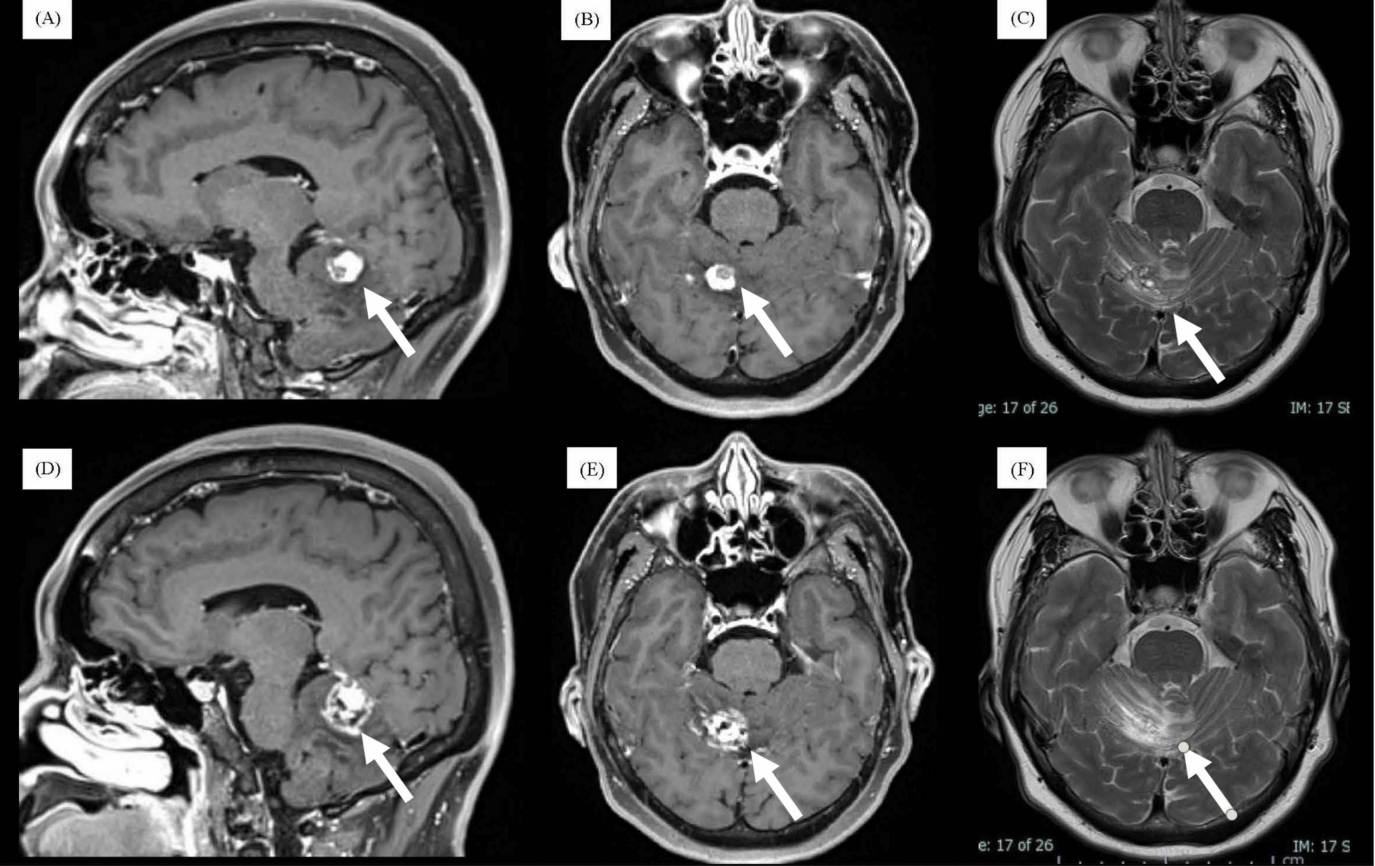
Pre-SRT (A-C) and post-SRT (D-F) MRI images (A-C) Pre-SRT MRI, showing a sagittal gadolinium-enhanced image (A), axial gadolinium-enhanced image (B), and axial T2-weighted image (C). A tumor with gadolinium enhancement is observed on the superior surface of the right cerebellum. (D-F) Post-SRT MRI (4 months later), showing a sagittal gadolinium-enhanced image (D), axial gadolinium-enhanced image (E), and axial T2-weighted image (F). SRT: stereotactic radiotherapy; MRI: magnetic resonance imaging
